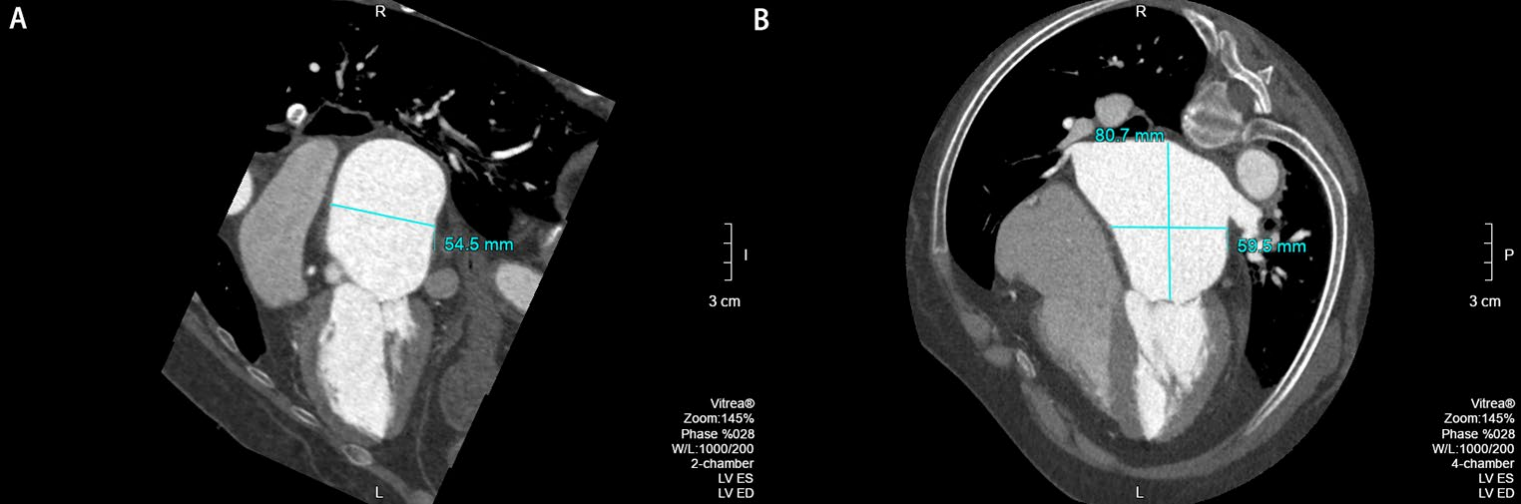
A: The anteroposterior diameter of the LA measured at the apical two-chamber plane, on the MPR image. B: Measurement of the long diameter and transverse diameter of LA at the apical four-chamber plane on the MPR image
